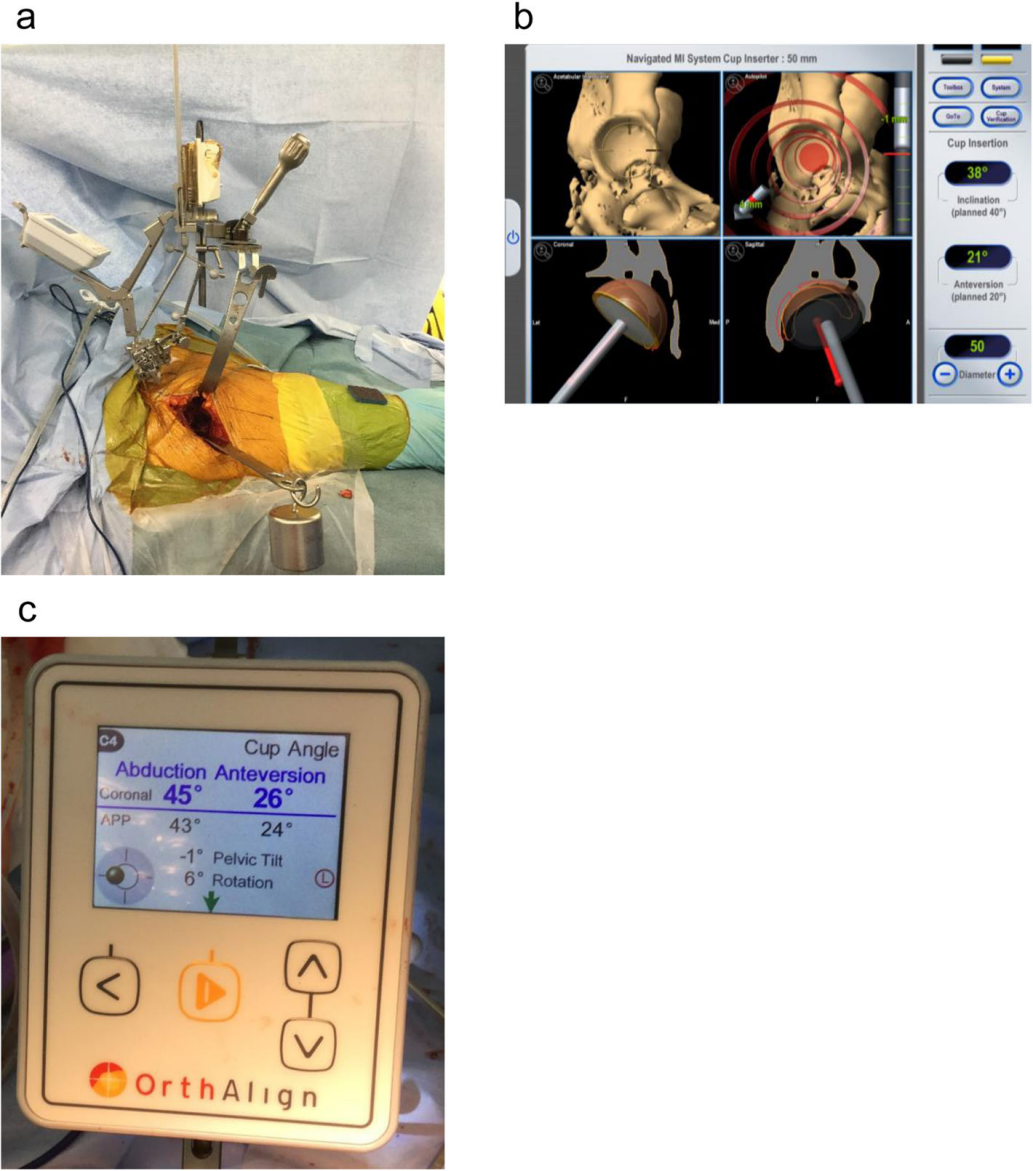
Total hip arthroplasty was performed in each patient with simultaneous CT- and accelerometer-based navigation (a). Operative inclination and operative anteversion were displayed on the CT navigation system (b), and radiographic inclination and radiographic anteversion were displayed on the accelerometer-based system (c). Pelvic tilt and pelvic rotation were also displayed simultaneously on the accelerometer-based system
